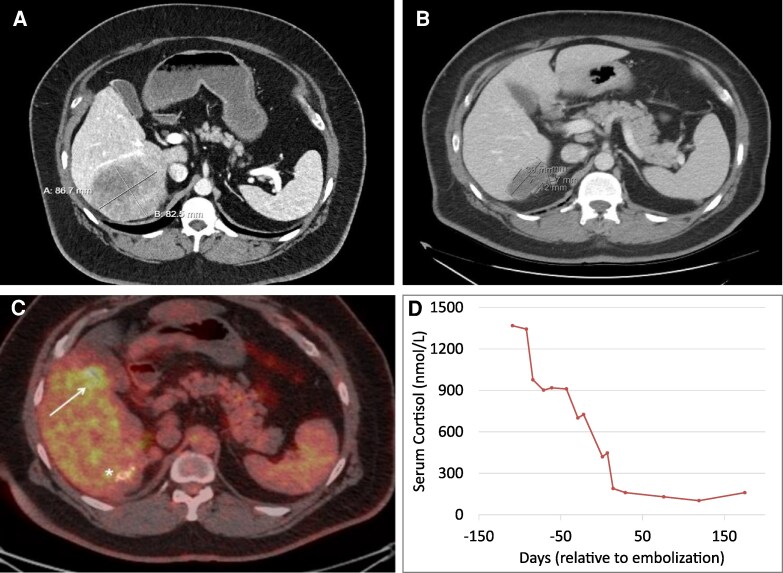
Response to hepatic embolization in case 1. Axial contrast-enhanced computed tomography images demonstrating a large centrally located hepatic metastasis prior to (A) and 7 months after (B) yttrium-90 embolization. The mass measured 8.7 × 8.3 cm pretreatment (A), with a marked reduction to 3.0 × 1.2 cm post-treatment (B). A follow-up 18F-FDG PET/CT (C) showed postradioembolization changes in segment 6 (*) and a new 4.4 cm hypermetabolic lesion in segment 5 near the gallbladder fossa (white arrow), along with new suprarenal nodules. Longitudinal serum cortisol trajectory following hepatic embolization procedure demonstrating sustained biochemical response following Y-90 embolization in Case 1 (D).
